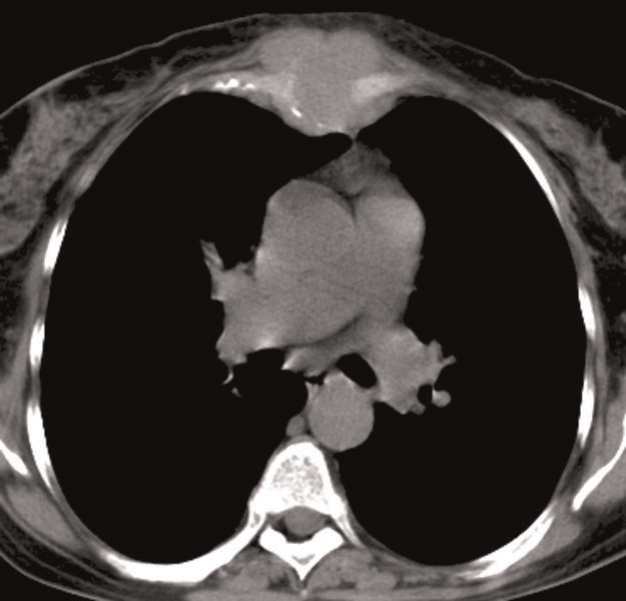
Thoracic CT scan showing soft-tissue mass in the sternum.
